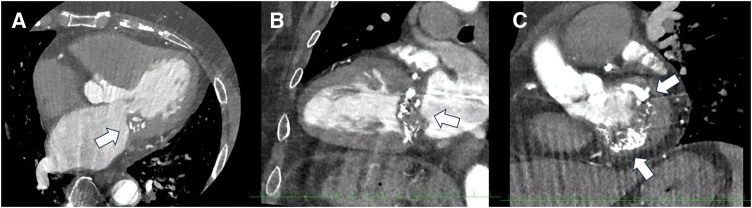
Cardiac CT. (A and B) The posterior leaflet of the mitral valve exhibits a tumor-like appearance with calcification (arrow). (C) A tumor-like appearance with calcification (arrow) was extending from the anterior commissure to the posterior commissure.
